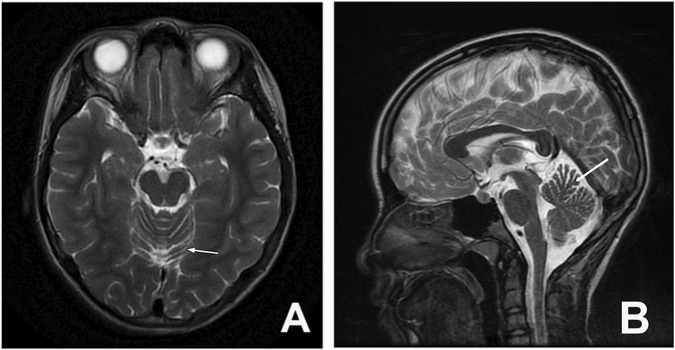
Cranial MRI: Arrow indicates mild atrophy of the anterior cerebellar lobe, no significant changes observed in the brainstem and upper cervical spinal cord ((A) transverse section; (B) sagittal section).
